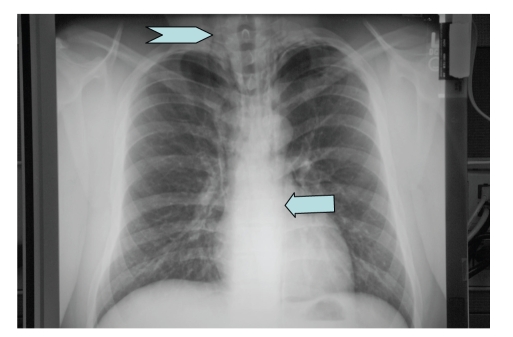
Initial chest X-ray showed subcutaneous emphysema, with air tracking into the neck area bilaterally (chevron), and a para-aortic air stripe of the left (arrow).
